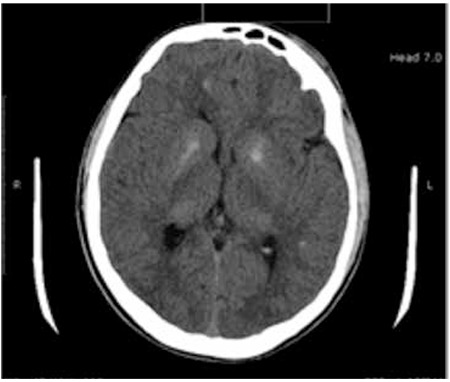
Multiple calcifications in bilateral centrum semiovale, corona radiata and basal ganglions were shown by cranial computed tomography
